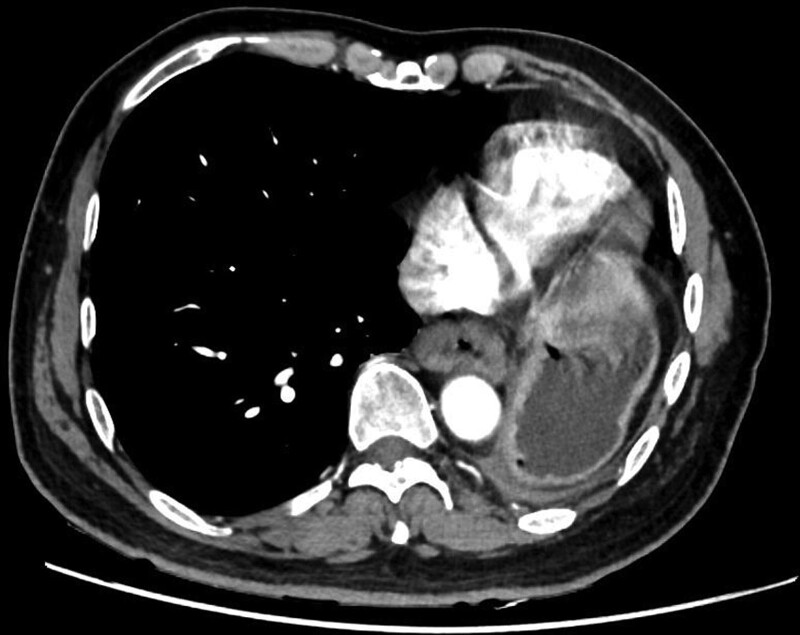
CT mediastinum window revealed a neoplasm, which was located in lower thoracic esophagus, measuring 5 × 3 cm in size as white arrow indicated. CT = computed tomography.
